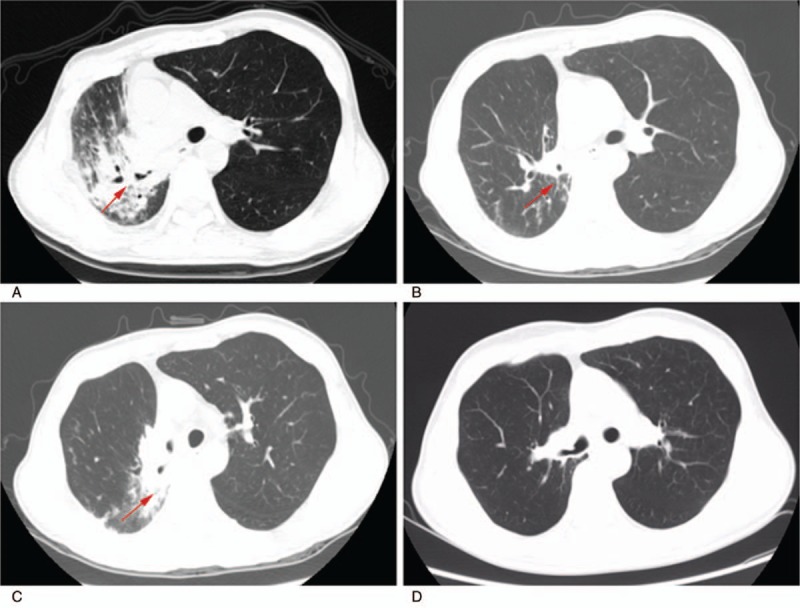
Chest computed tomography of the lung tumor patient after repeat radiotherapy. (A) At 1 month after repeat radiotherapy, consolidation with air bronchogram in the hilum of the right lung and ground-glass densities in the right lobe were observed. (B) After intravenous methylprednisone, the patchy shadow on the chest radiograph nearly disappeared. (C) At 1 month after tapering oral prednisolone, a consolidated shadow was observed again inside the irradiated volume. (D) At 24 months after FP/Salm maintenance therapy, complete resolution was observed in the right lung.
